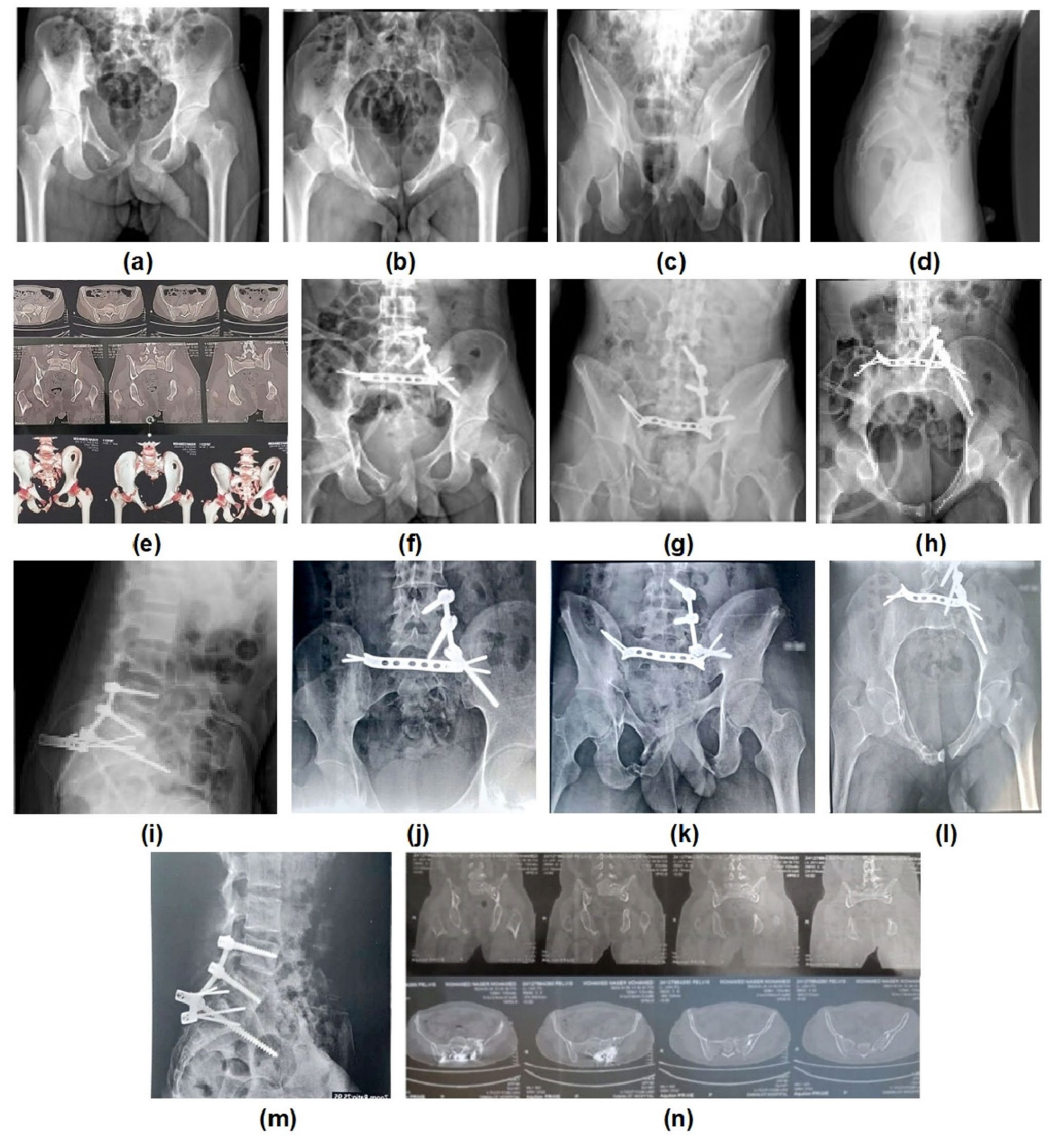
Preoperative (a, b, c, and d) radiographs of a 22-year-old man, (e) Computed tomography showing AO C1 sacral fracture, (f, g, h and i) Postoperative radiographs, (j, k, l and m) Follow up radiographs 1 year postoperatively, (n) CT showing fracture union
